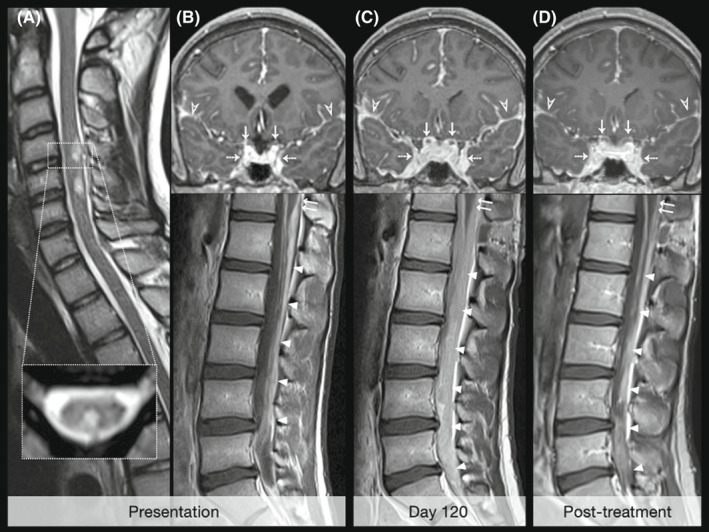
MRI features on initial presentation (A, B) and temporal evolution pre‐treatment (B, C) and post‐treatment (D). Small, T2 hyperintense pseudocystic lesions are present within the dorsal aspect of the cervical spinal cord on sagittal (A) and axial (A, inline) T2‐weighted images of the cervical spine. Coronal (B, top pane) post‐contrast T1‐weighted MR images of the brain demonstrate extensive nodular thickening and enhancement of the intracranial leptomeninges, including the Sylvian fissures (open arrowheads), basal cisterns, parasellar regions (dashed arrows), falx cerebri and middle cranial fossae. Suprasellar leptomeningeal disease encases the optic nerves on both sides (arrows). Serial coronal T1‐weighted images of the brain (B‐D, top row) and sagittal post‐contrast T1‐weighted images of the lumbosacral spine (B‐D, bottom row) obtained at initial presentation (B), 4 months after presentation (C) and 2 weeks following initiation of MEK‐inhibitor (D) demonstrate radiological progression over time (B, C) and subsequent response to treatment (D). On presentation, there was extensive intracranial leptomeningeal disease with associated communicating hydrocephalus (B, top pane). In the lumbosacral spine, leptomeningeal thickening and enhancement lining the conus medullaris (double arrows in B, bottom pane) and cauda equina (arrowheads in B, bottom pane) was evident. Four months later and following shunt insertion (C), the ventricles were decompressed but the leptomeningeal disease in the brain and spine had worsened (labels in C, top pane). Leptomeningeal thickening and enhancement lining the conus medullaris and cauda equina (double arrows and arrowheads in C, bottom pane) now completely fills the thecal sac and encases the neural structures. Two weeks after initiation of treatment (D), there was a significant improvement in the bulk of the intracranial (labels in D, top pane) and intraspinal enhancing leptomeningeal disease (labels in D, bottom pane).
